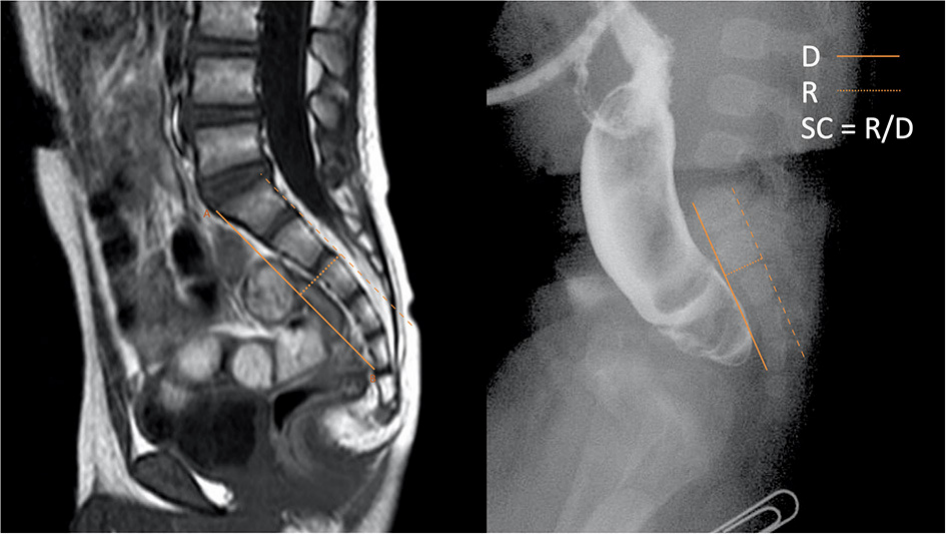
SC measurement in MRI and x-ray.
